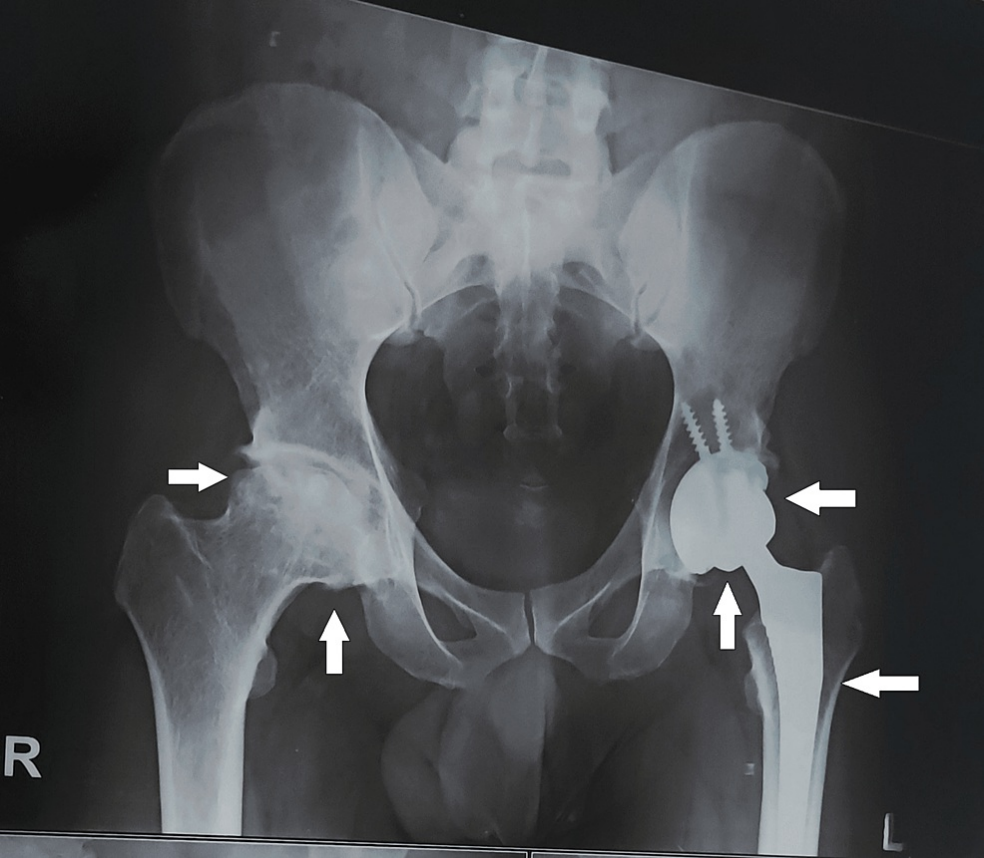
Anteroposterior view of an X-ray of the pelvis showing the destruction of the femoral head on the right side and partial hip replacement on the left side.
